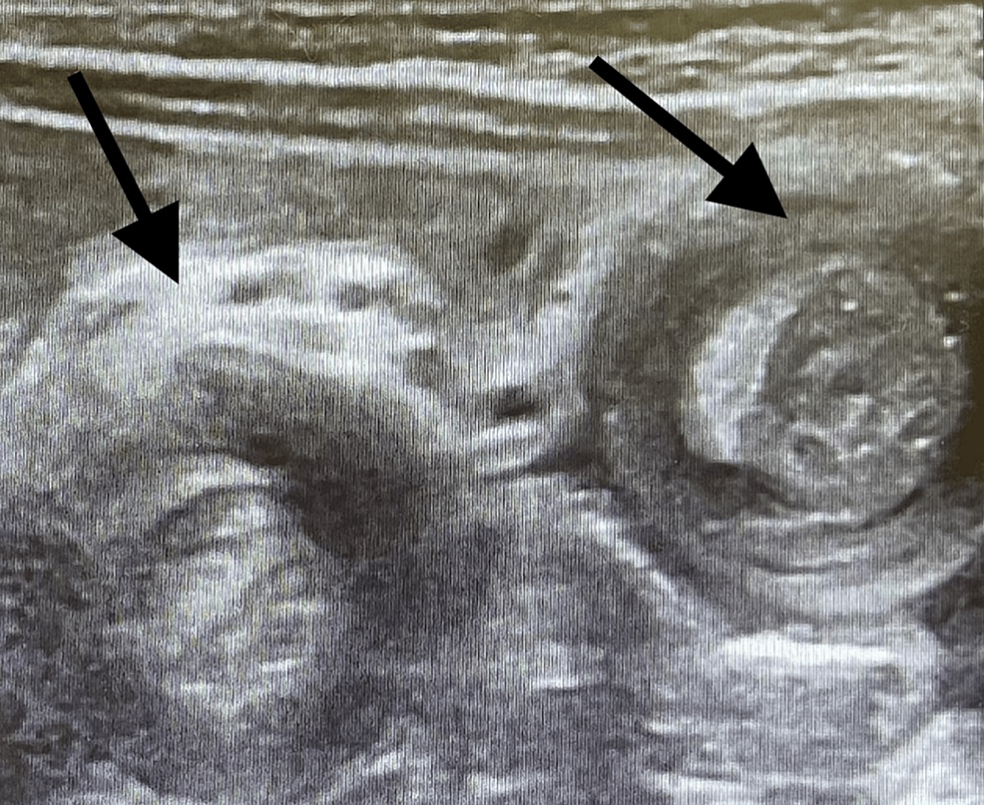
Day 2 abdominal ultrasound image showing the double target sign in the right upper quadrant
